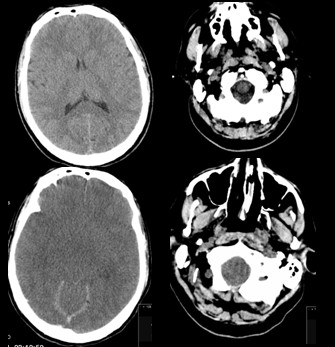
CT- scan: cerebral swelling in intracranial hypertension (Cerebral malaria).
